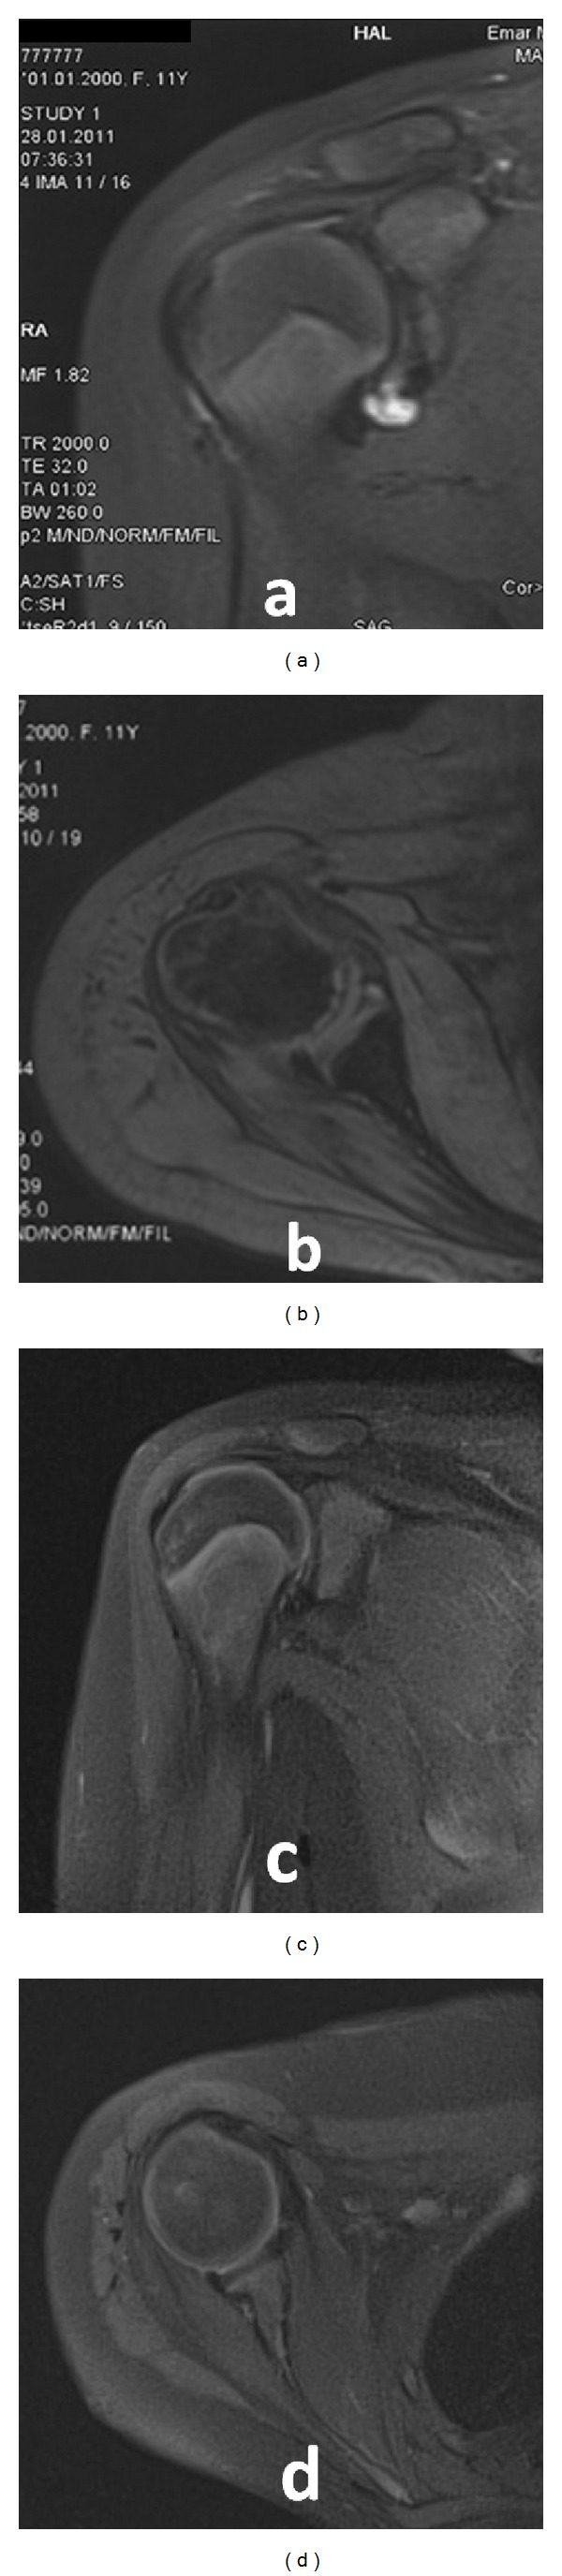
Preoperativecoronal (a) and axial (b) MRI views of capsulolabral separation. Postoperative one-year coronal (c) and axial (d) MRI views of capsulolabral repair.
